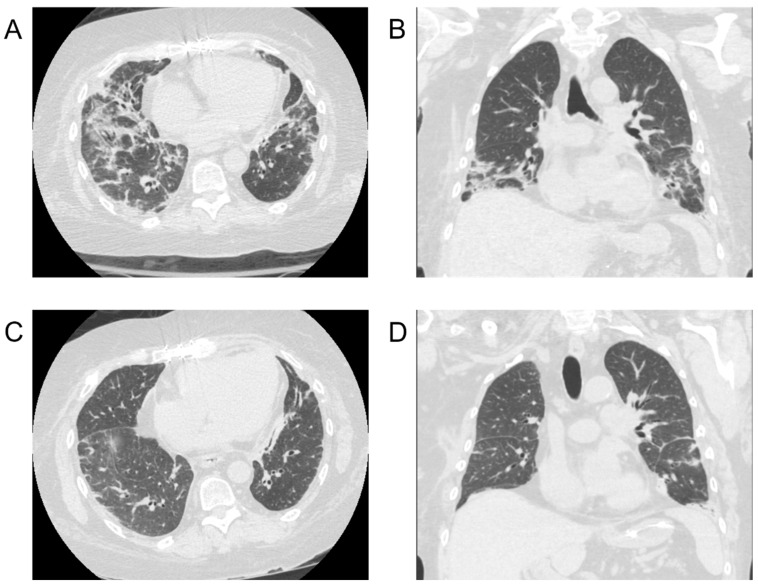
Case 4. CT scans of SARS-CoV-2 infection. (A) Axial image 18 days after diagnosis; bilaterally moderate patchy ground glass opacities in the lower lobes. (B) Coronal image 18 days after diagnosis; bilaterally moderate patchy ground glass opacities in the lower lobes. (C) Axial image 52 days after diagnosis demonstrating exceptional recovery of lung parenchyma. (D) Coronal image 52 days after diagnosis demonstrating exceptional recovery of lung parenchyma.
